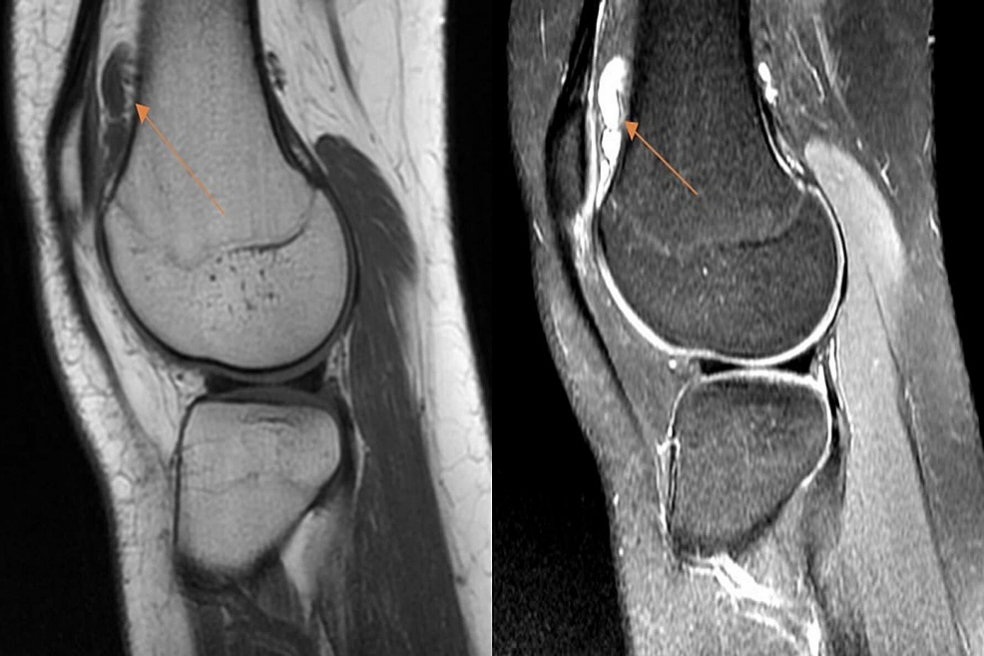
Hypointense lesion on T1-weighted MRI sequences and hyperintense lesion on T2-weighted MRI sequence show a cystic lesion within the pre-femoral fat.
